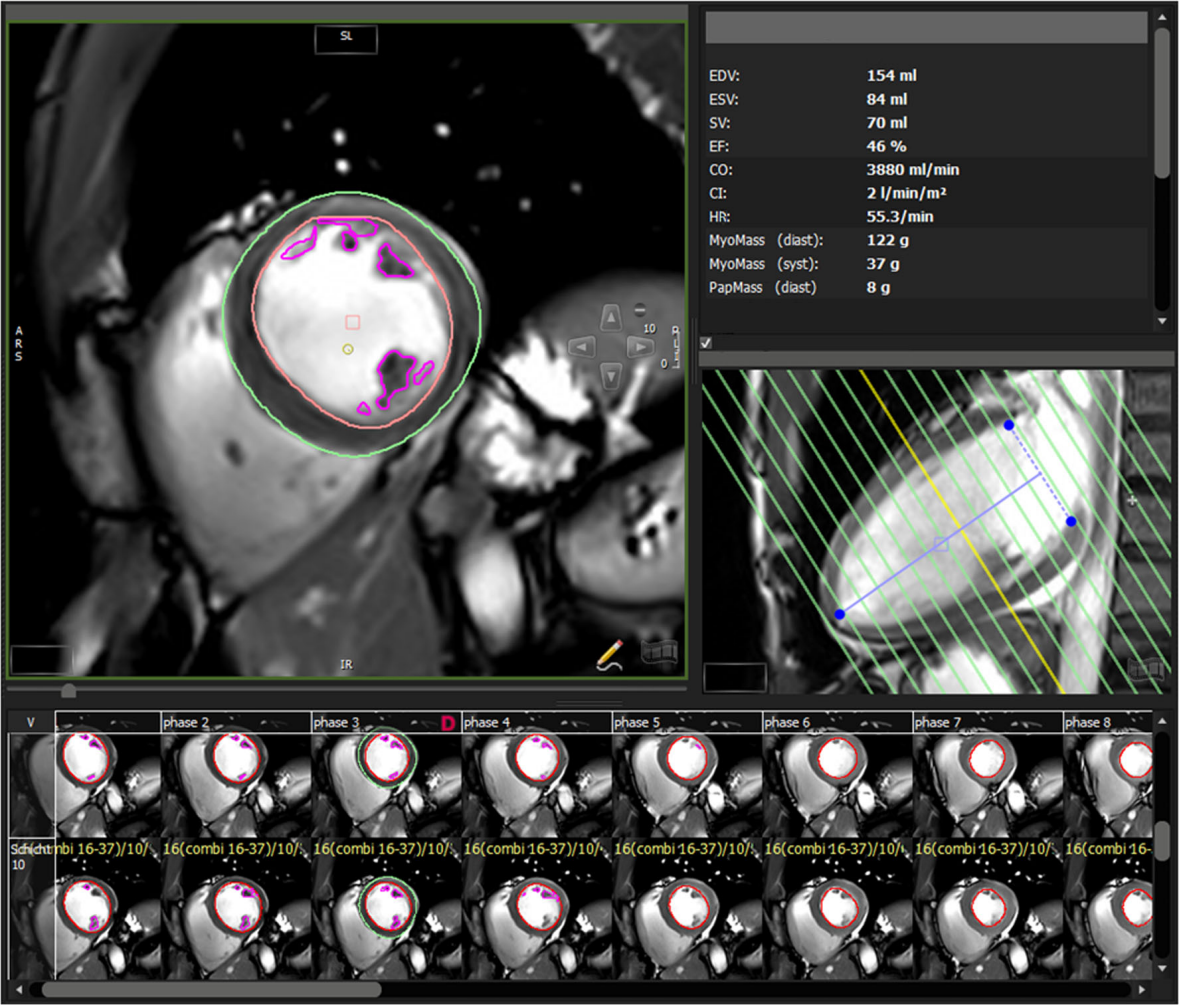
Evaluation of short axis cine MR images using cmr42. *MRI data evaluation of a simultaneously acquired PET/MR data set in a patient with a previous non-cardiac PET/CT examination:* Delineation of the epicardial and endocardial contours in the short axis orientation in the end-diastole (phase 3) is shown in the left upper frame. The image locator in the two-chamber view is depicted in the left mid-centre. The volumes of the ventricle and the calculated ejection fraction are depicted on the top right centre of the image. Note that papillary muscles were determined separately (*violet segmentation*)
